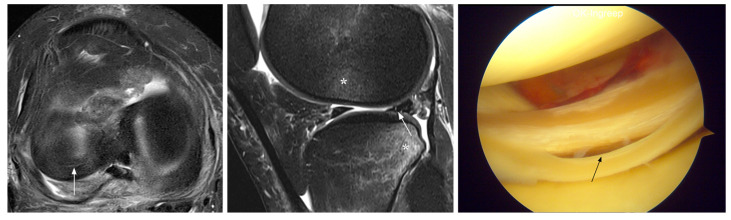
Interpretation false-negative MRI diagnosis (patient 19). MRI shows a tear at the posterior horn of the lateral meniscus that was missed at prospective reading and confirmed by arthroscopy (arrows). Also note lateral bone contusions due to acute ACL injury (*).
